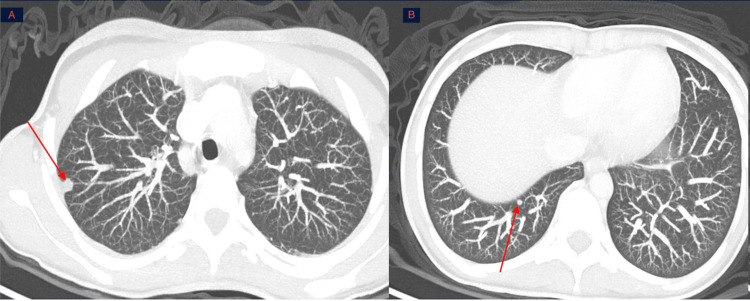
Thoracic CT scan in lung window, axial slices with MIP reconstructions, showing pulmonary nodules and micronodules, the largest measuring 7.3 × 6 mm (A) and 4.4 × 3 mm Thoracic CT scan in lung window (axial slices with MIP reconstructions) demonstrates a dense pulmonary micronodule in the dorsal segment of the right upper lobe, measuring 7.3 × 6 mm (red arrow, A), as well as another micronodule in the right posterobasal segment, measuring 4.4 × 3 mm (red arrow, B). CT: computed tomography, MIP: maximum intensity projection
